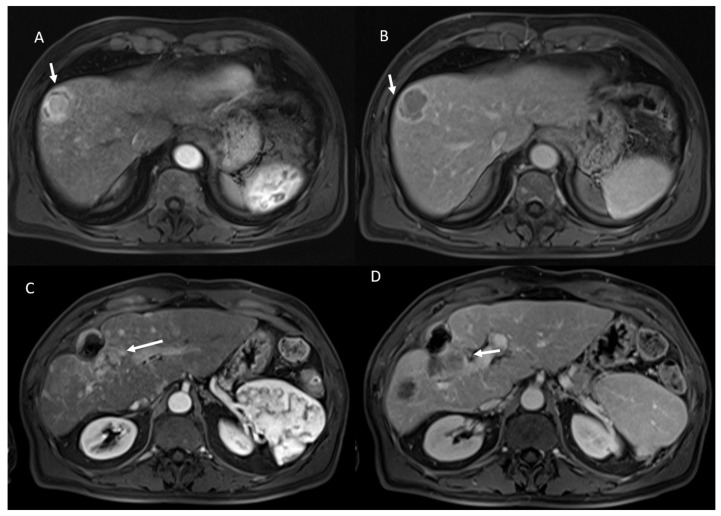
HCC on VIII hepatic segment: arrow shows APHE in arterial phase (A), with wash out and capsule appearance in portal phase (B). Post-treatment MRI: arrow shows APHE (C) and wash-out appearance in portal phase (D) in viable lesions.
